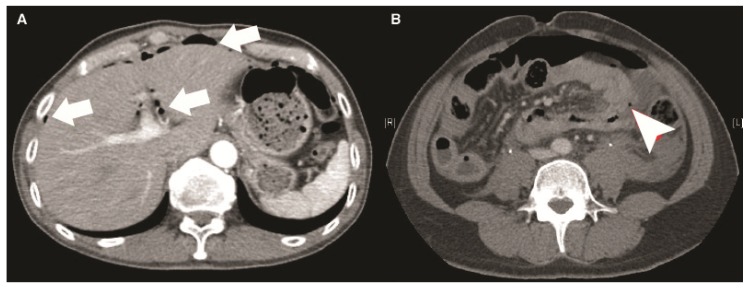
(A) The axial computed tomography presented multiple extraluminal air (arrows). (B) The abdominal computed tomography revealed a small extraluminal air bubble with focal fluid (arrowhead).
